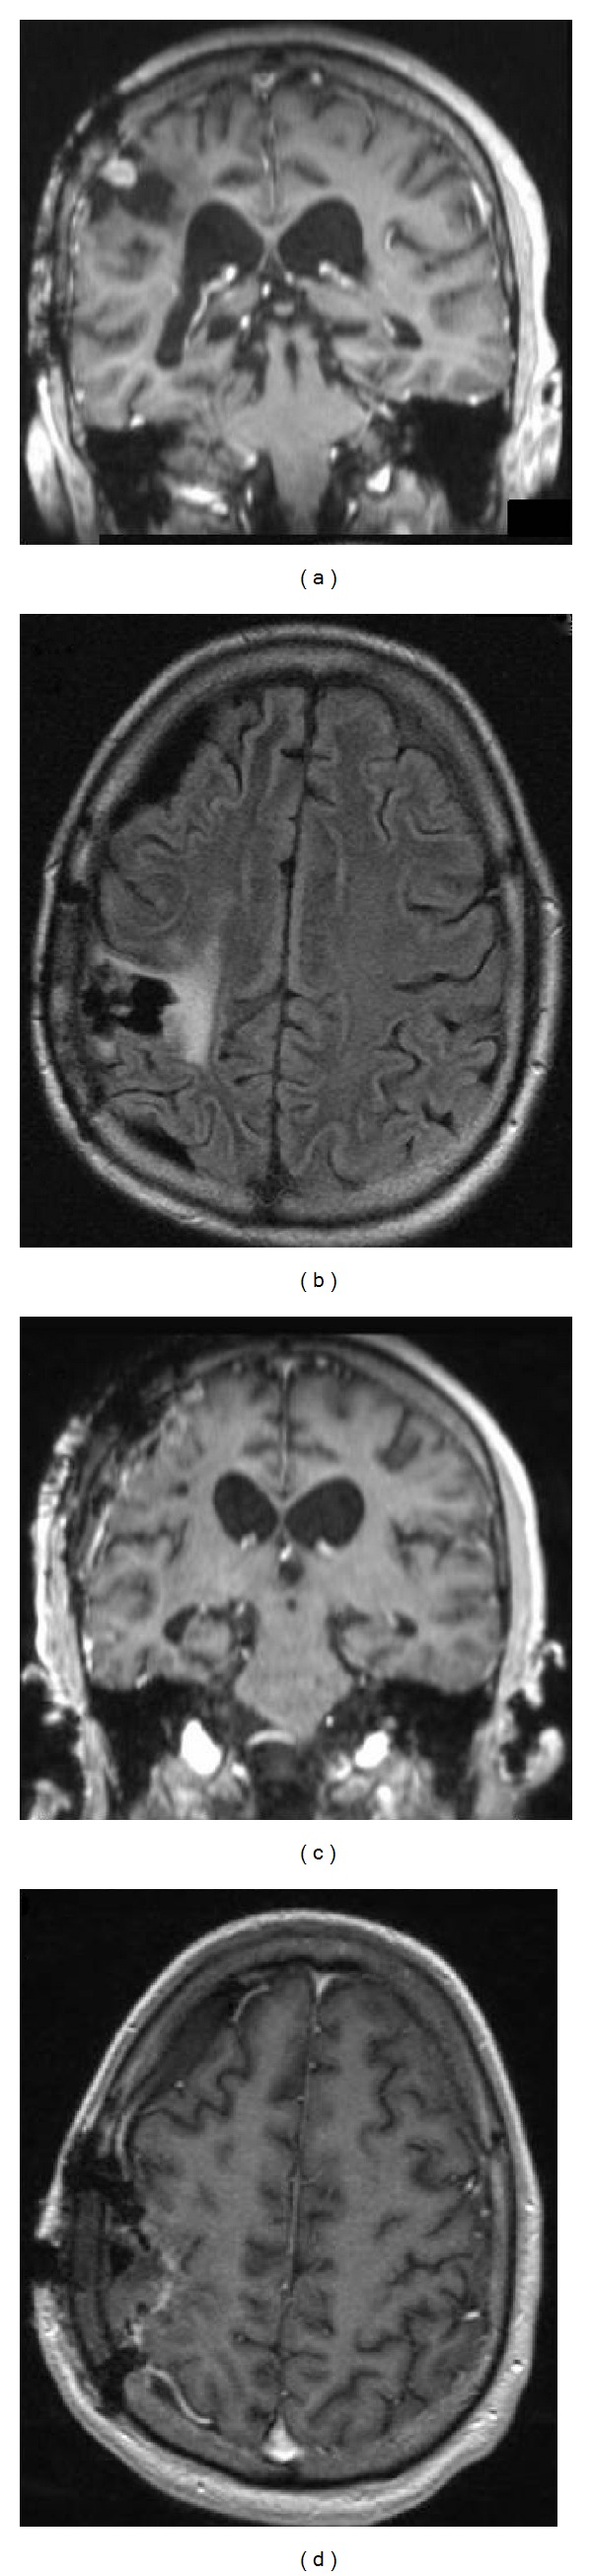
((a),(b)) Preoperative axial flair MRI and T1 coronal MRI showing a recurrent new 7 mm nodular area of enhancement at the superior aspect of the surgical cavity associated with the dura. ((c),(d)) Postoperative axial flair MRI and T1 coronal MRI showing resection of the lesion.
